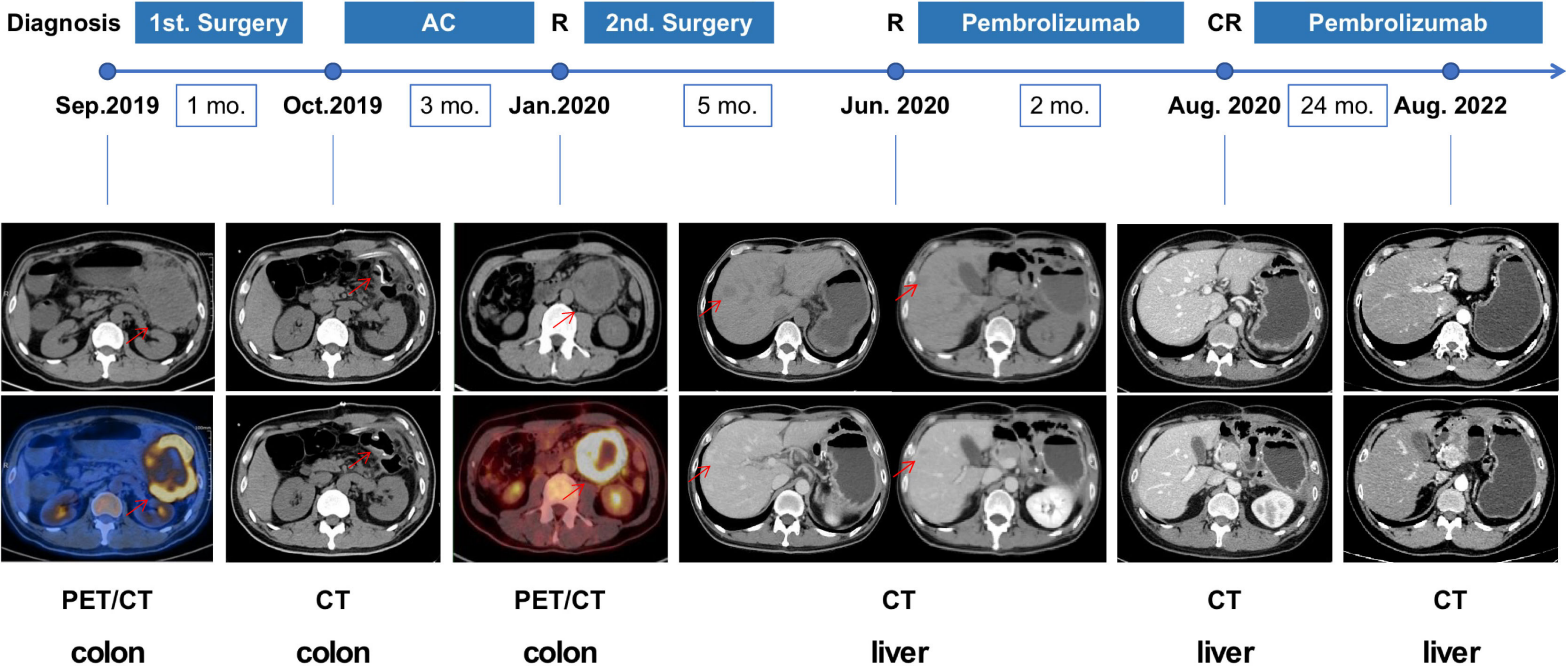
The course depicting the treatment process and radiological evaluation from 2019 to 2022. The patient has received pembrolizumab since June 2020, achieving clinical CR after 2 months and has remained in CR until now. AC, adjuvant chemotherapy; CR, complete response; R, recurrence; CT, computed tomography; PET/CT, positron emission tomography/computed tomography.
